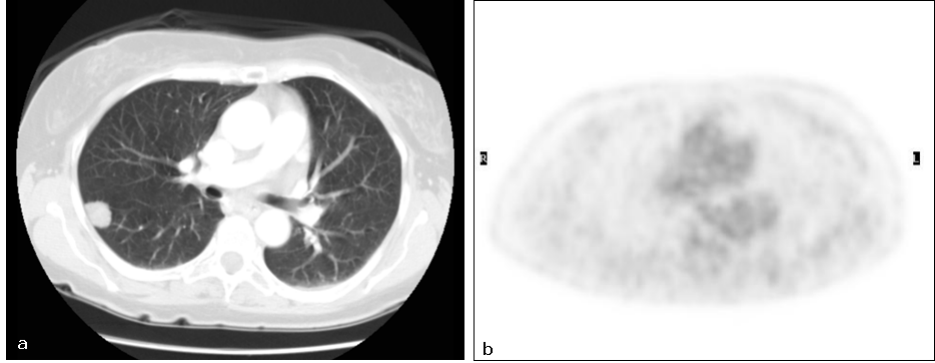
Primary lung cancer. Patient #14. Lung cancer in the right lower lobe as detected by (a) CT (TP CT) and (b) PET/CT did not reveal increased FDG uptake (FN PET/CT).
